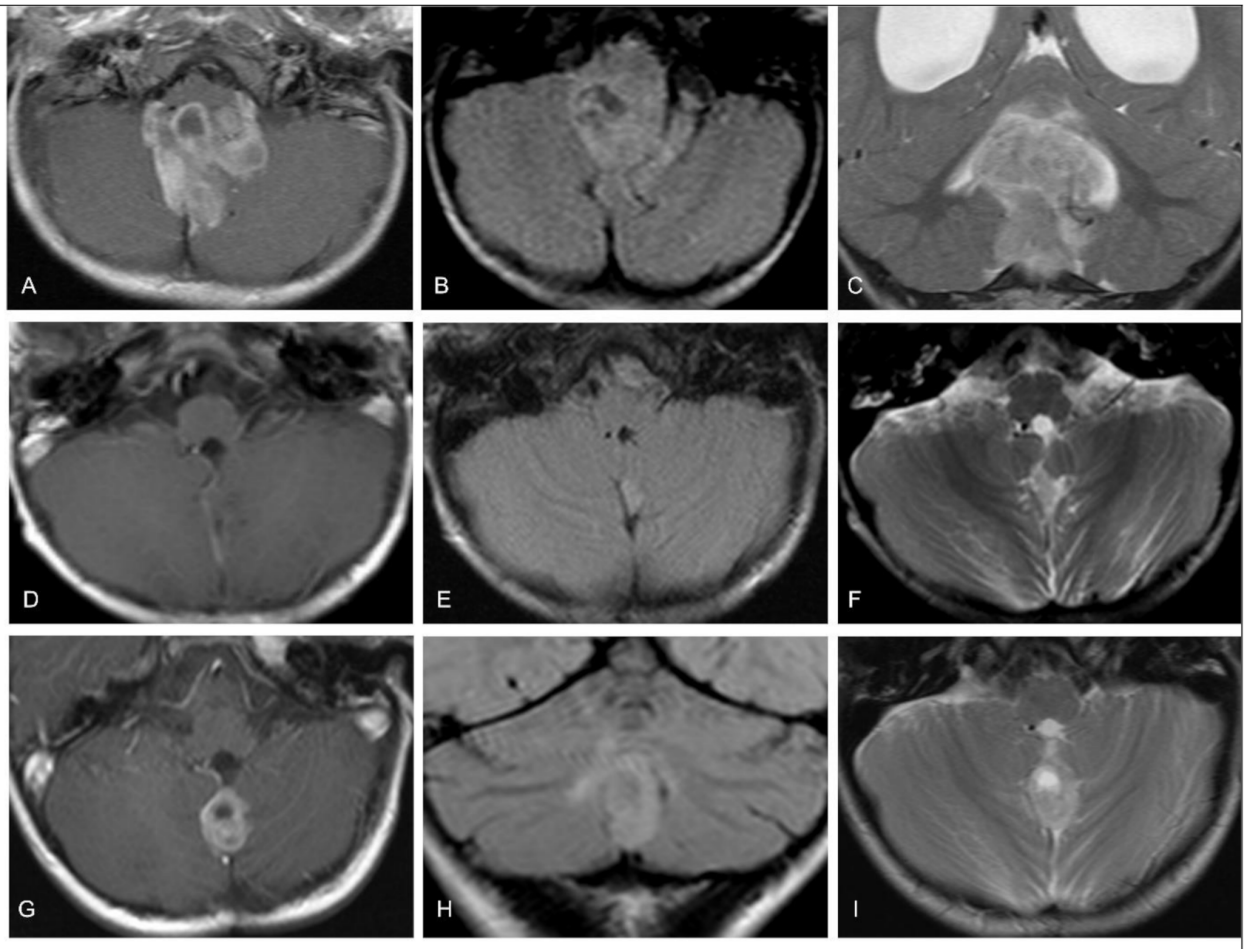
A 1-year-old girl with a posterior fossa ependymoma. (A-C) At diagnosis. The primary tumor showed strong contrast-enhancement (A) and inhomogeneous hyperintense signal intensity on T2-weighted and FLAIR images (B, C). (D-F) Local recurrent tumor at time-point 1, two years after total resection. The new lesion is best seen on T2-FLAIR (E) and T2 (F). T2-signal was similar compared to the primary tumor but there was lack of contrast-enhancement (D). (G-I) Local recurrent tumor at time-point 2, 22 months after time-point 1. The recurrent tumor now shows signal intensities resembling the primary tumor
